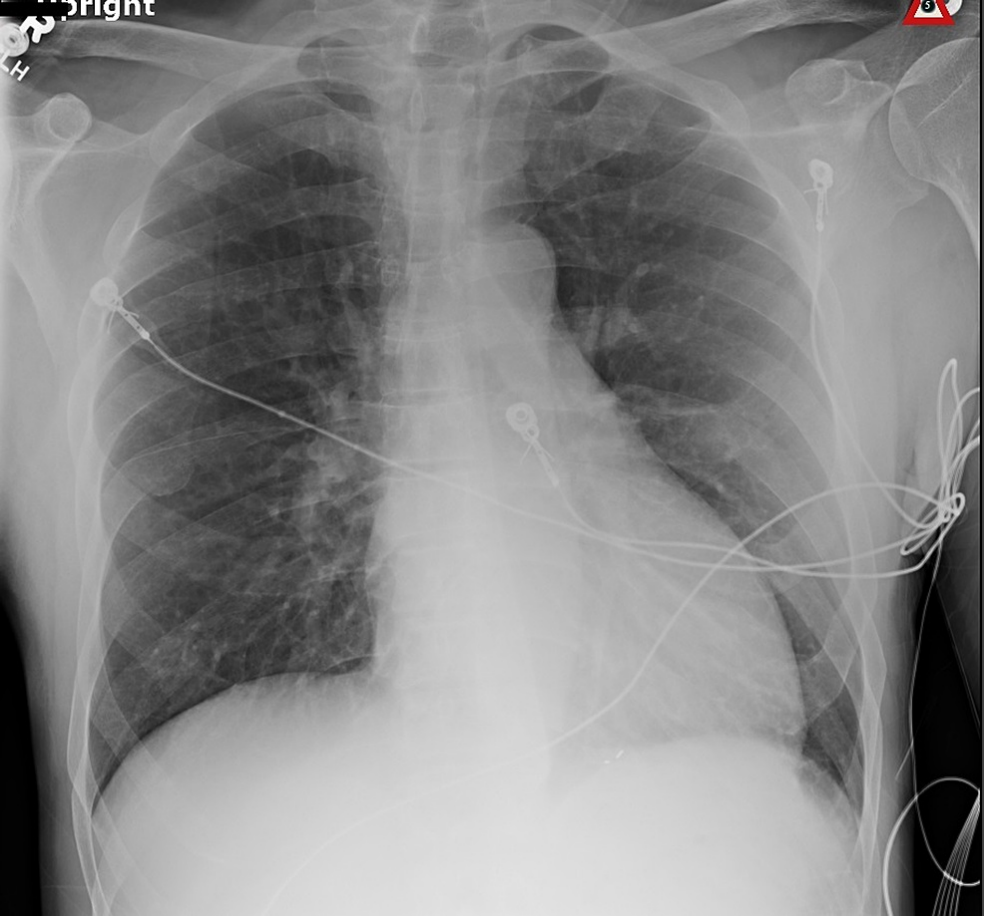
Chest X-ray findings. A single anteroposterior portable chest X-ray was obtained. No acute intrathoracic processes were observed.
